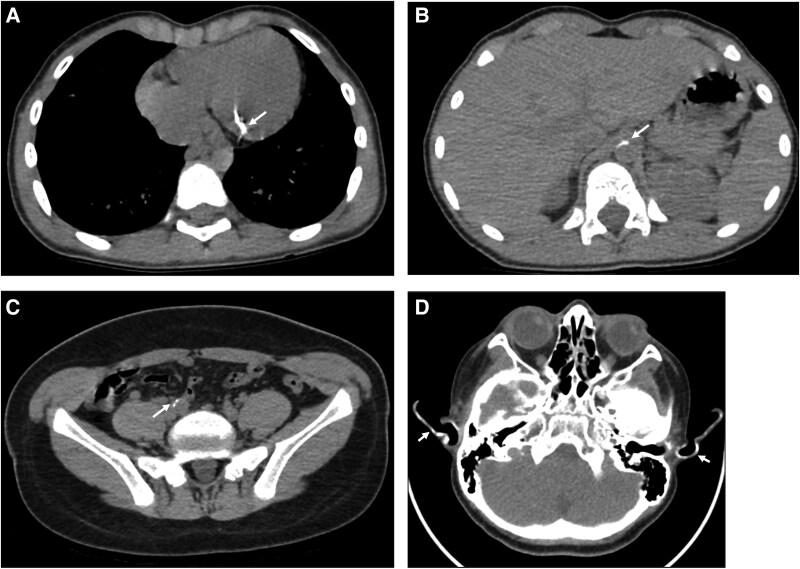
CT imaging in siblings with ENPP1-related generalized arterial calcification of infancy (GACI). Panel (A) demonstrates calcification of the left circumflex coronary artery in sibling 2. Panel (B) shows calcification at the origins of the superior mesenteric and celiac arteries in sibling 2. Panel (C) shows calcification at the origin of the right renal artery in sibling 1. Panel (D) demonstrates calcification of the auricular cartilage in sibling 1.
